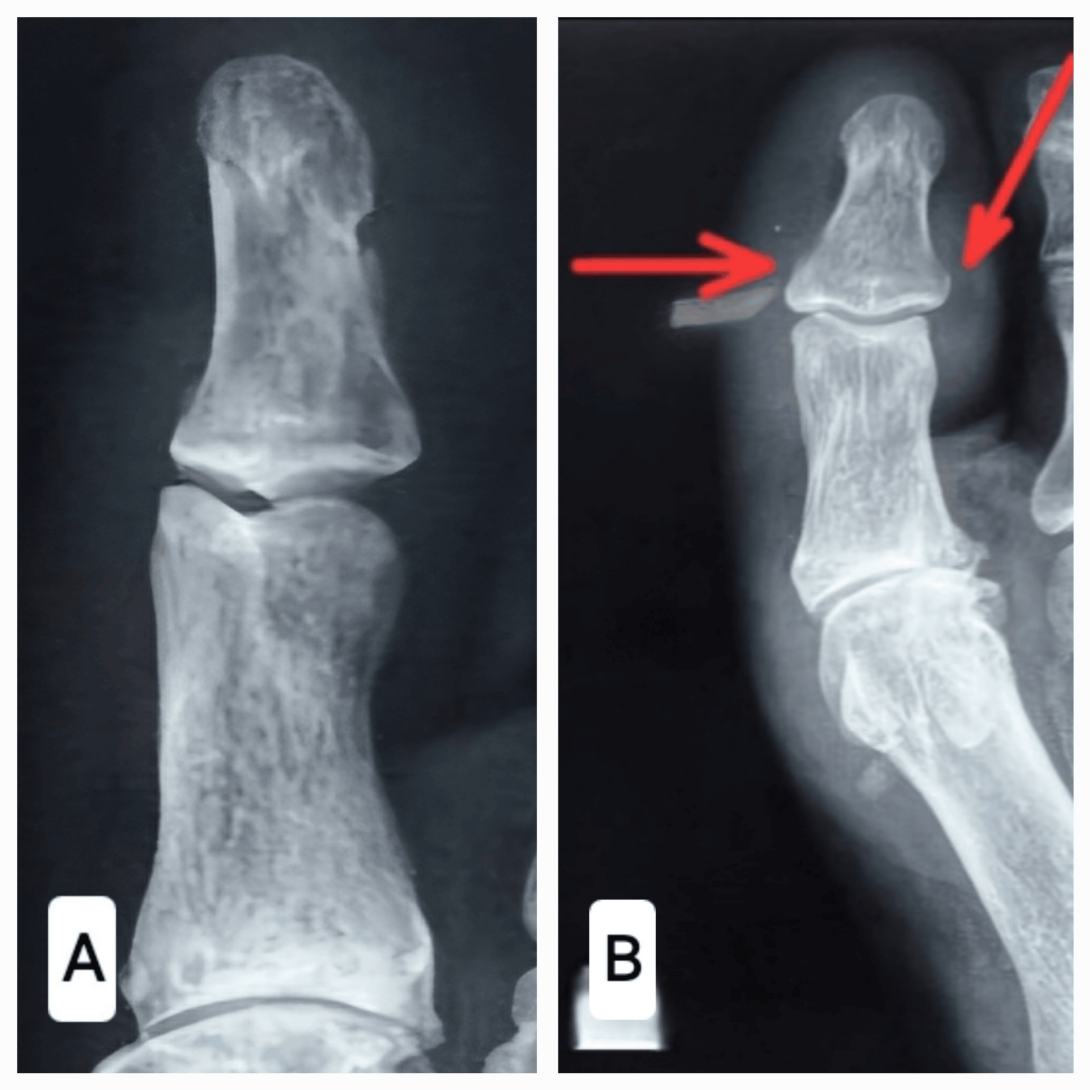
The plain radiograph of the affected great toe. A: The proximal and distal phalanx in the great toe appears to be normal. B: The distal phalanx of the affected toe shows post-traumatic cortical breach (red arrow).
